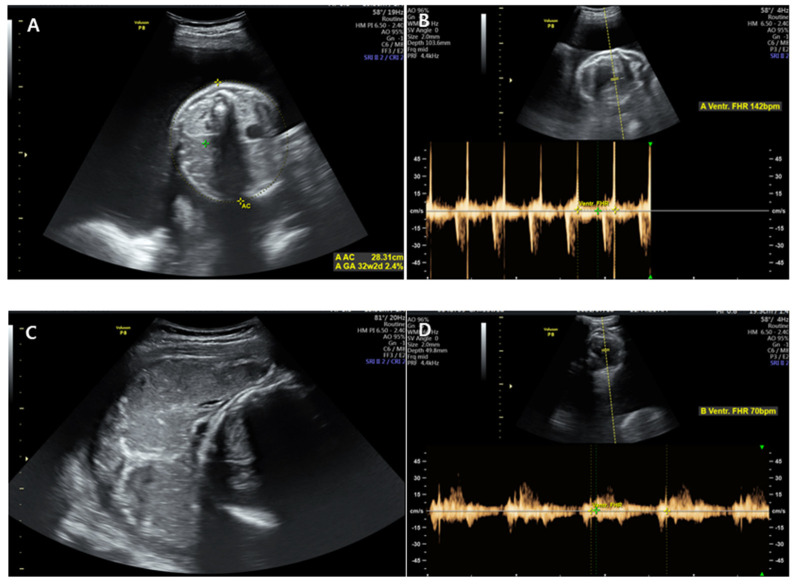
Ultrasonography was performed at admission. (A,B) showed the first fetus located on the left side of the mother. The fetal heart rate was 140–160 beats/min with good fetal motion, and amniotic fluid volume index (AFI) was more than 30 cm, showing polyhydramnios. (C,D) showed the second fetus located on the right side of the mother with breech presentation. The heart rate was 70–80 beats/min, showing a consistent bradycardia.
